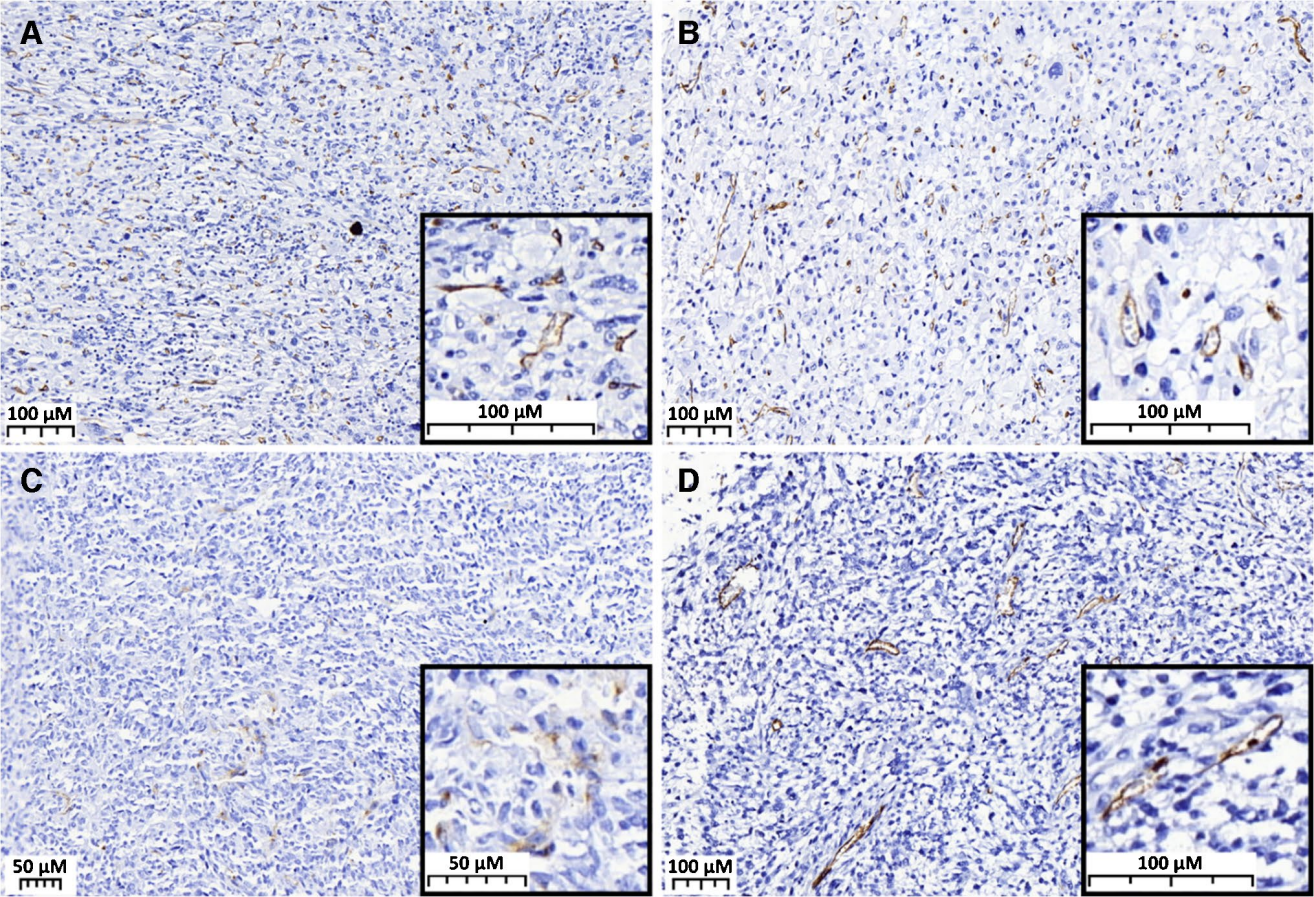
PSMA expression in neovasculature of soft tissue sarcomas. A MPNST showing intense and diffuse expression of PSMA. B Undifferentiated pleomorphic sarcoma with PSMA index 2. C Synovial sarcoma with PSMA index 1. D Leiomyosarcoma with diffuse and intense expression of PSMA in the neovasculature
